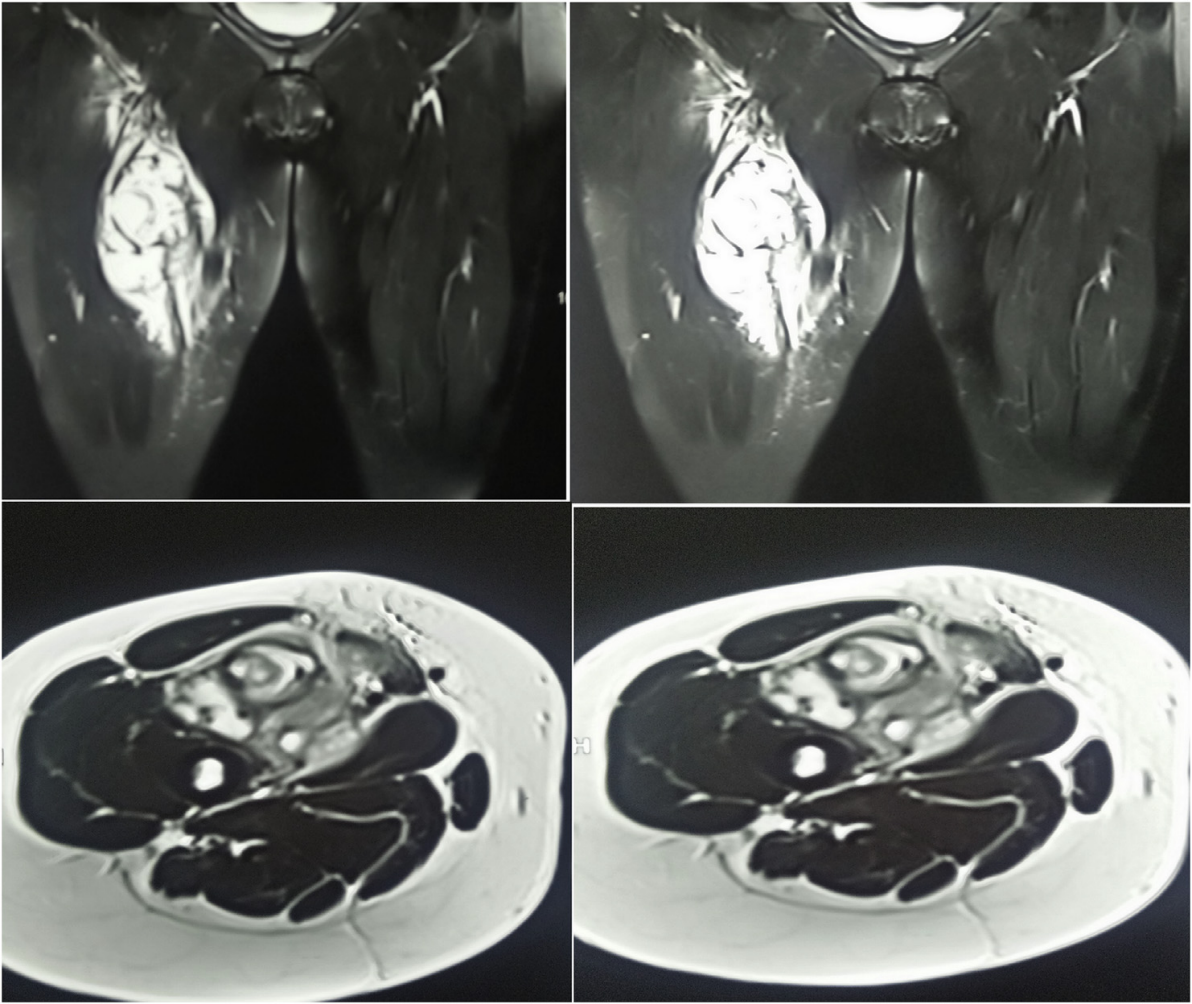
T2-weighted MRI study of the thigh showing a multi-loculated cystic lesion in the right thigh with multiple internal septations.
